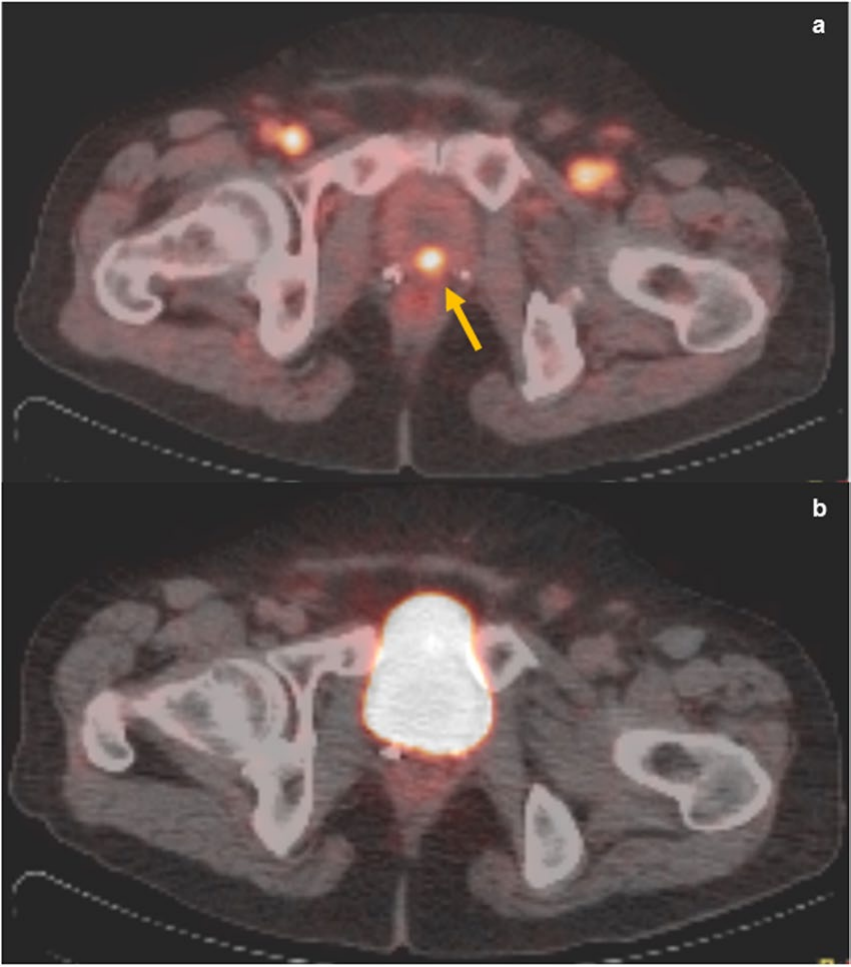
73 year old male presenting for prostate cancer staging. (a) The prostate gland shows a focus of increased activity in its center (arrow) with SUV max 4.2 at 3 minutes. Radiotracer activity in the inguinal vessels is also visible. Early PET/CT imaging helped detect tumor lesion that was obscured by radiotracer activity in the bladder on standard imaging at 60 minutes post-injection (b).
